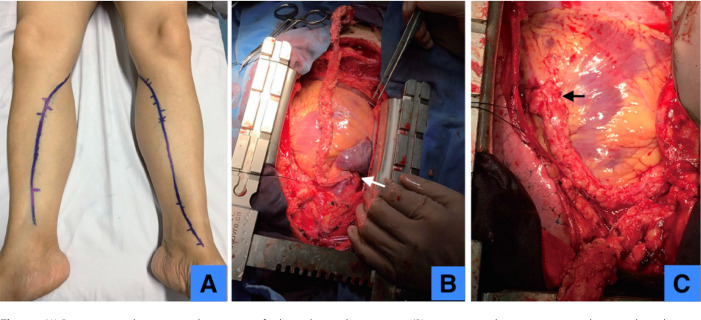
(A) Preoperative ultrasonography mapping for lower leg saphenous
